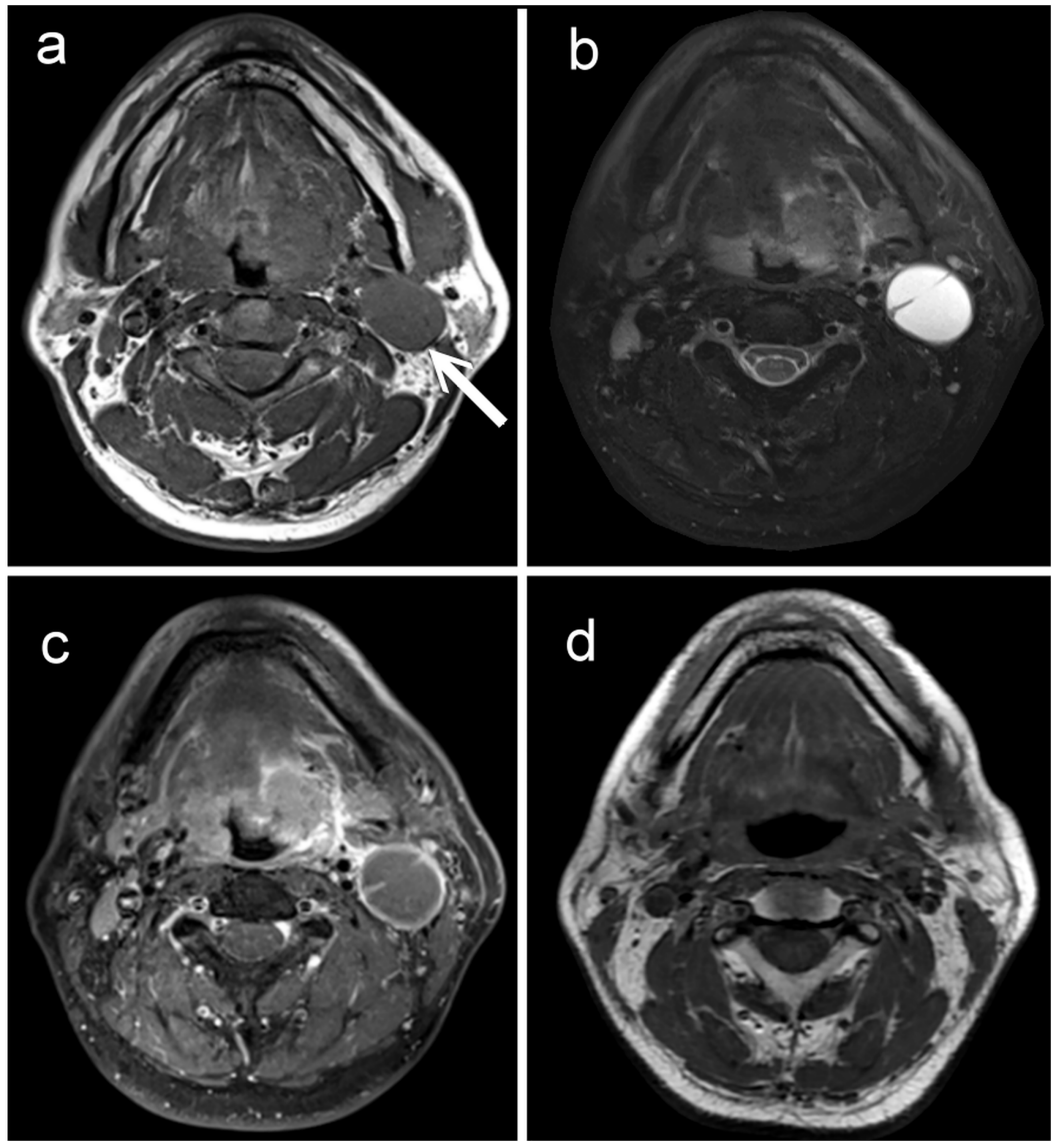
A 46-year-old male patient with left tongue base oropharyngeal squamous cell carcinoma and cystic nodal metastasis. (A) T1-weighted, (B) fat-suppressed T2-weighted, and (C) contrast-enhanced fat-suppressed T1-weighted MRI images show an enlarged cystic lymph node (arrow) in the left neck level IIA with a thin, well-defined smooth margin, and central homogeneous fluid content. (D) T1-weighted MRI three months after completion of chemoradiotherapy shows regression of the neck node. The patient was disease-free during three years of clinical and imaging follow-up.
